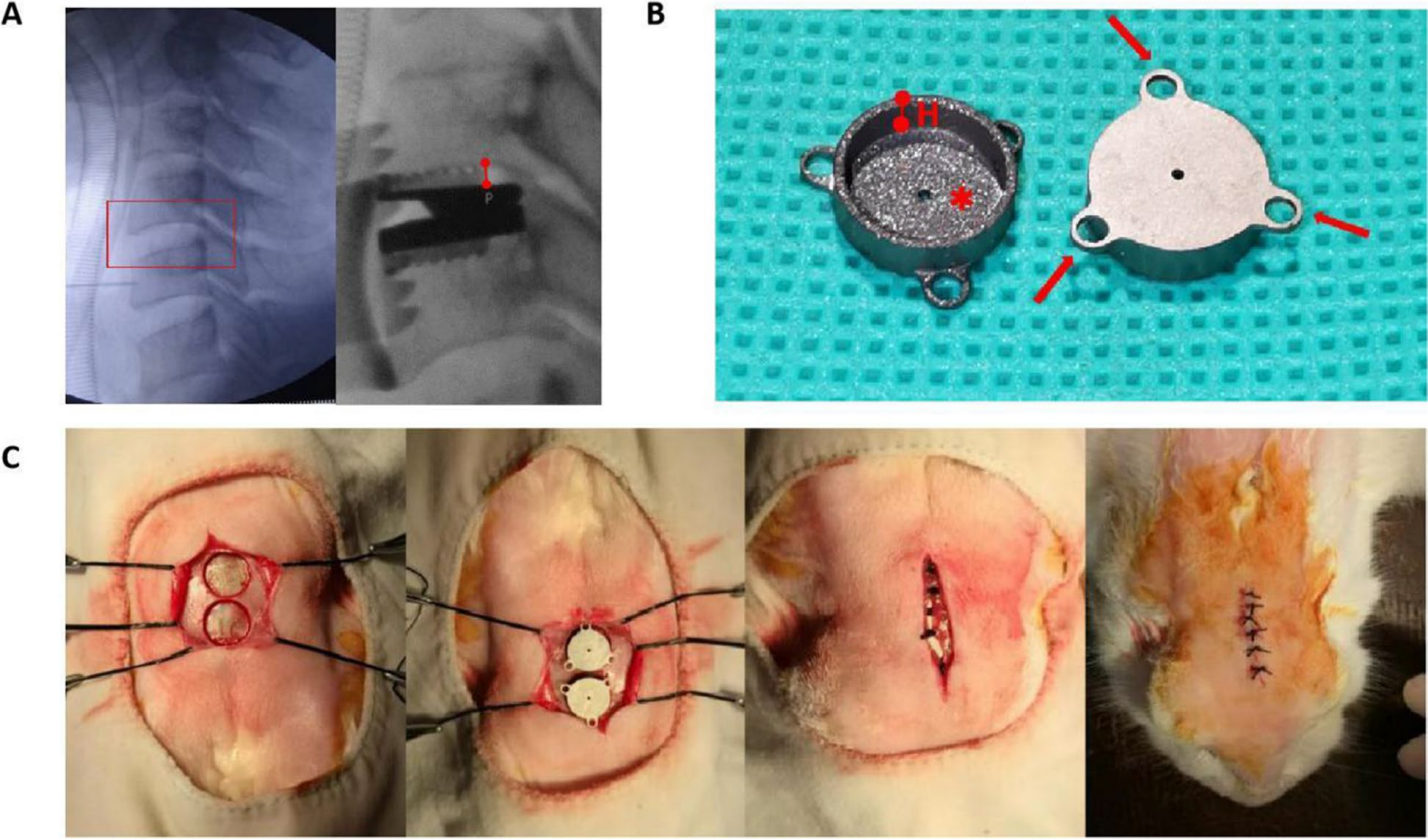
A Left: Intraoperative lateral X-ray image of cervical spine before insertion of prosthesis (Red box: Operational segment with an obvious doom-shape inferior endplate). Right: Intraoperative lateral X-ray image of operational segment after insertion (Red line segment: Max distance between endplate and footprint). B The custom-made titanium implant used in the present study (Arrow: Rings designed as the fixture of implant in biomechanical testing; H: The depth of the inner cavity is designed as 2 mm; *:Porous hydroxyapatite-coated inner surface. C Surgical procedures showing a sequential of exposure and drilling, insertion and closure in layers
